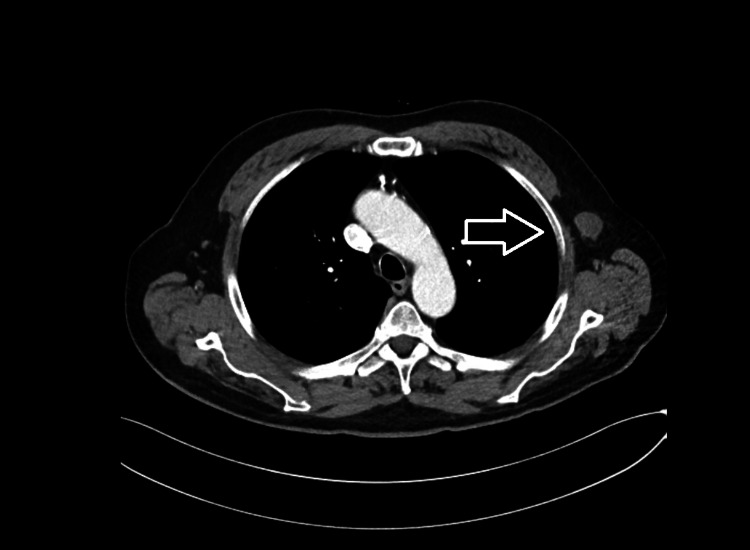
Axial view of chest CT scan with contrast demonstrating multiple left axillary and subpectoral lymph nodes, the largest of which measured 3.3 x 1.9 cm (white arrow).
